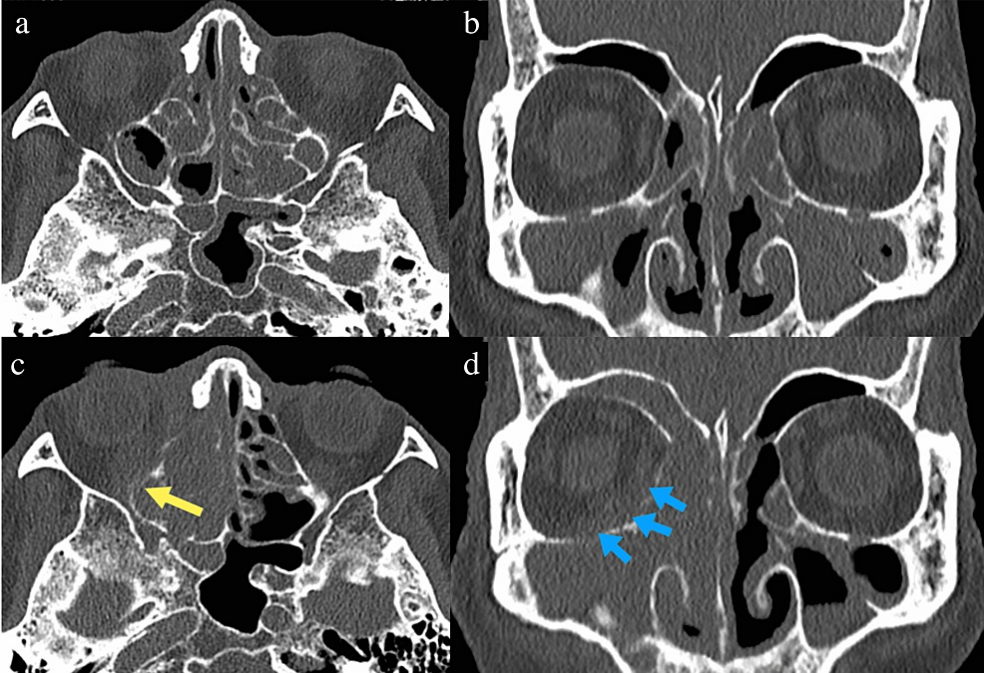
Images of computed tomography at the ages of 79 and 81 At age 81, compared with the computed tomography at age 79 (a-b), the intraorbital bony wall is obscured (c, yellow arrow). There is also an extension of the soft shadow within the orbit (d, blue arrow).
